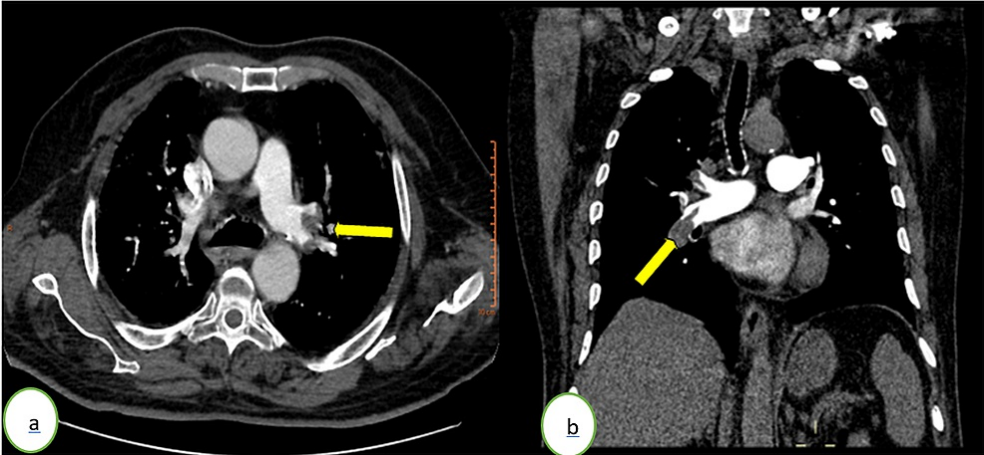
Computed tomography pulmonary angiography in (a) axial and (b) coronal windows showing a bilateral acute pulmonary embolism (yellow arrows) of the right pulmonary artery and a segmental branch of the left upper lobar branch
